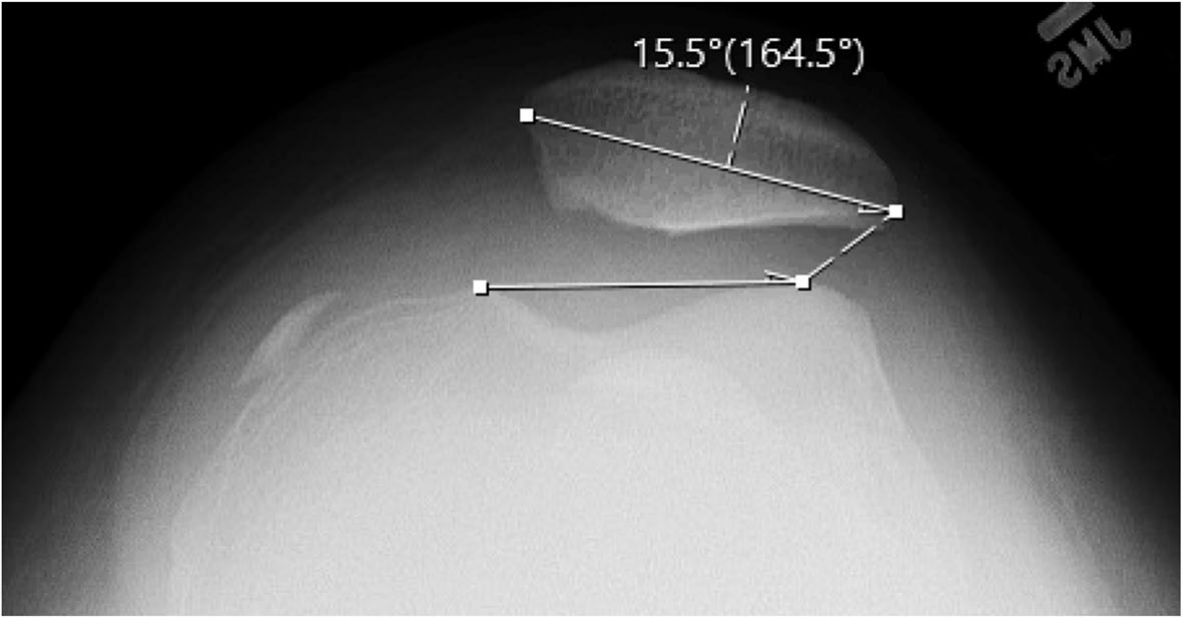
Patellar tilt as calculated on Merchant view radiograph
